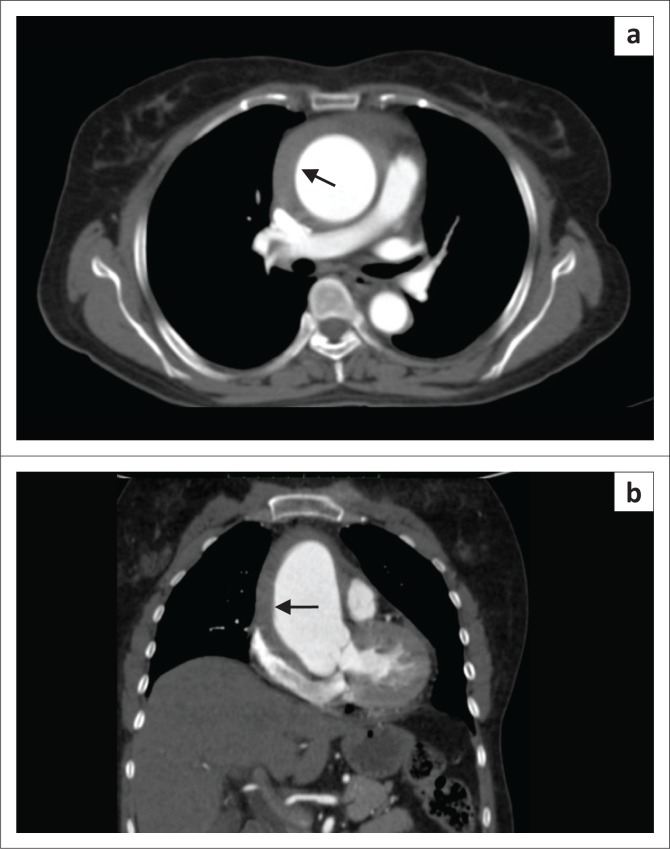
Type A intramural haematoma. Contrast-enhanced axial (a) and coronal (b) computed tomography scan showing a non-enhancing hypodense crescent-shaped area (arrow) compressing the normal contrast-enhanced aortic lumen.
